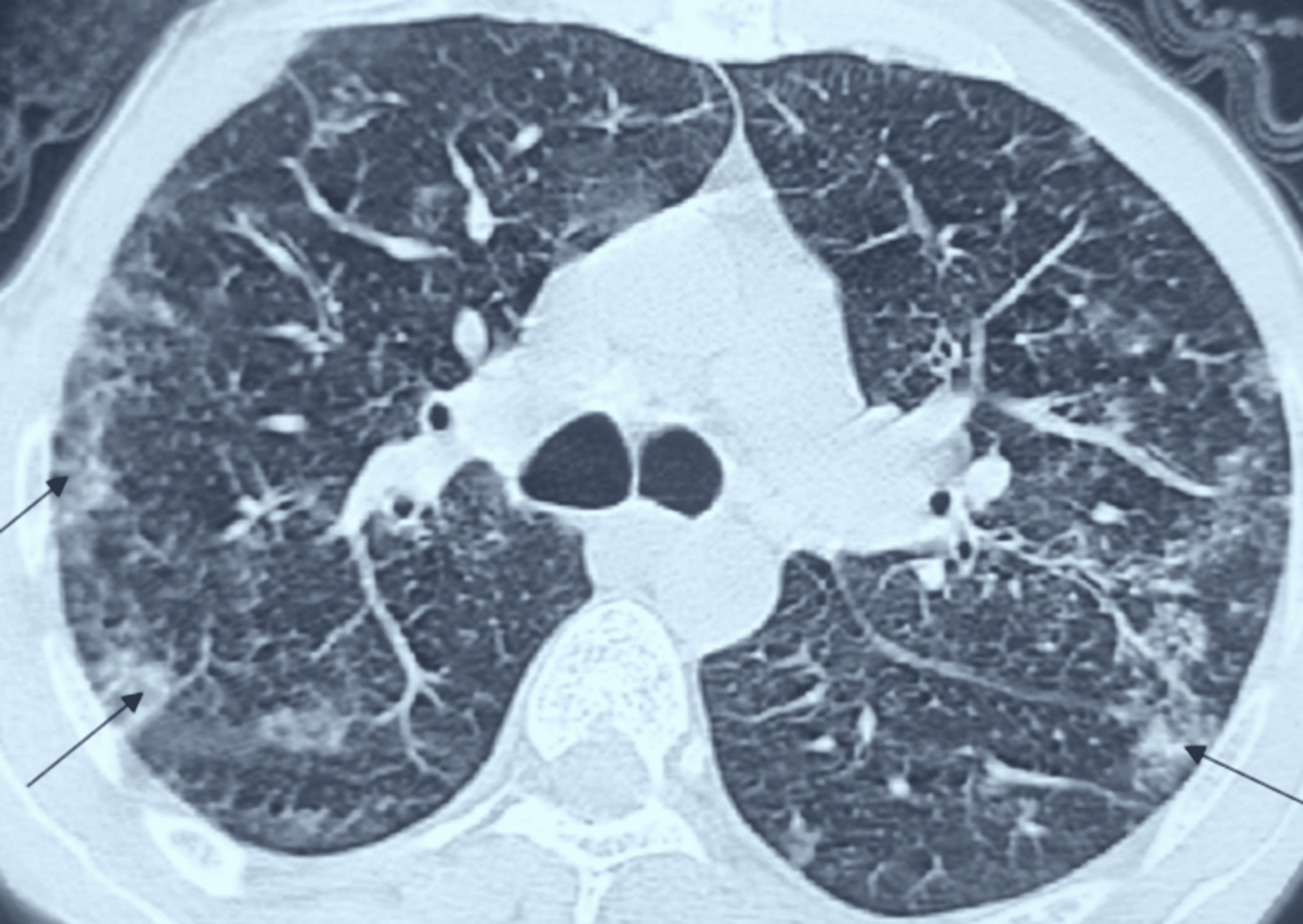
Representative axial chest CT slices showing bilateral peripheral ground-glass opacities (black arrows) CT: computed tomography
